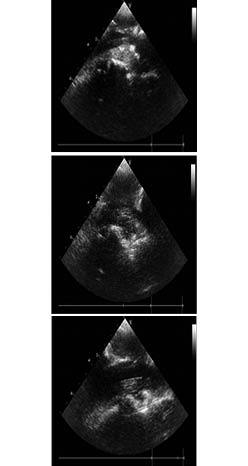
Intraoperative B-mode ultrasound.
